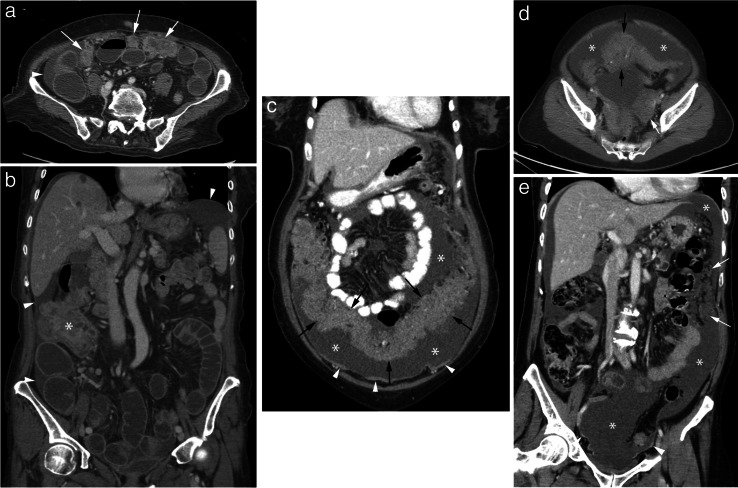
Case examples of carcinomatosis. a,b Axial and coronal CT images from a 61-year-old woman demonstrates centrally necrotic peritoneal nodules (arrows) and ascites (arrowheads) consistent with peritoneal carcinomatosis. The primary lesion was pathologically confirmed to be from the ascending colon (asterisk). c,d Coronal and axial CT images from a 54-year-old woman demonstrates a large quantity of ascites (asterisks), thick omental caking (black arrows) and plaque-like peritoneal thickening (arrowheads). The primary lesion was pathologically confirmed to be from the left ovary (white arrow). e Coronal CT image from a 58-year-old woman with adenocarcinoma of unknown primary. Note the large amount of ascites (asterisks), omental nodularity (arrows) and nodular and plaque-like peritoneal thickening (arrowheads). A primary lesion was not identified in this patient
